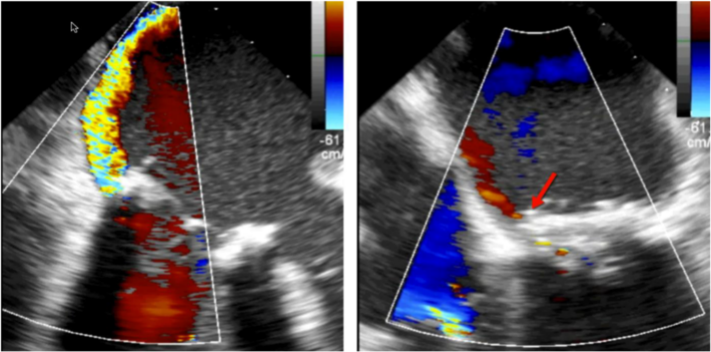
Trans-esophageal echocardiography with color Doppler. Left: Pre-procedure: Antero-medial mitral paravalvular leak associated with moderate-severe mitral regurgitation. Right: Post deployment of Amplatzer vascular plug (#red arrow) showing significant reduction of mitral regurgitation
